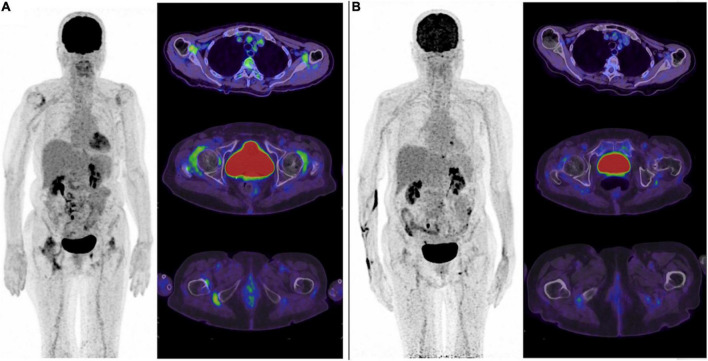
^18^F-FDG PET changes under treatment: typical but no current benefit over standard biomarkers. In this case of PMR, significant decrease of ^18^F-FDG uptake is observed in the targeted joints (here the scapular and pelvic girdles, ischial tuberosities) under treatment (A) baseline scan, and (B) after several lines of treatment). Although metabolic changes assessed with ^18^F-FDG are frequently observed, the clinical benefit of such imaging biomarker over standard clinical and biological biomarkers for disease monitoring remains to be defined.
